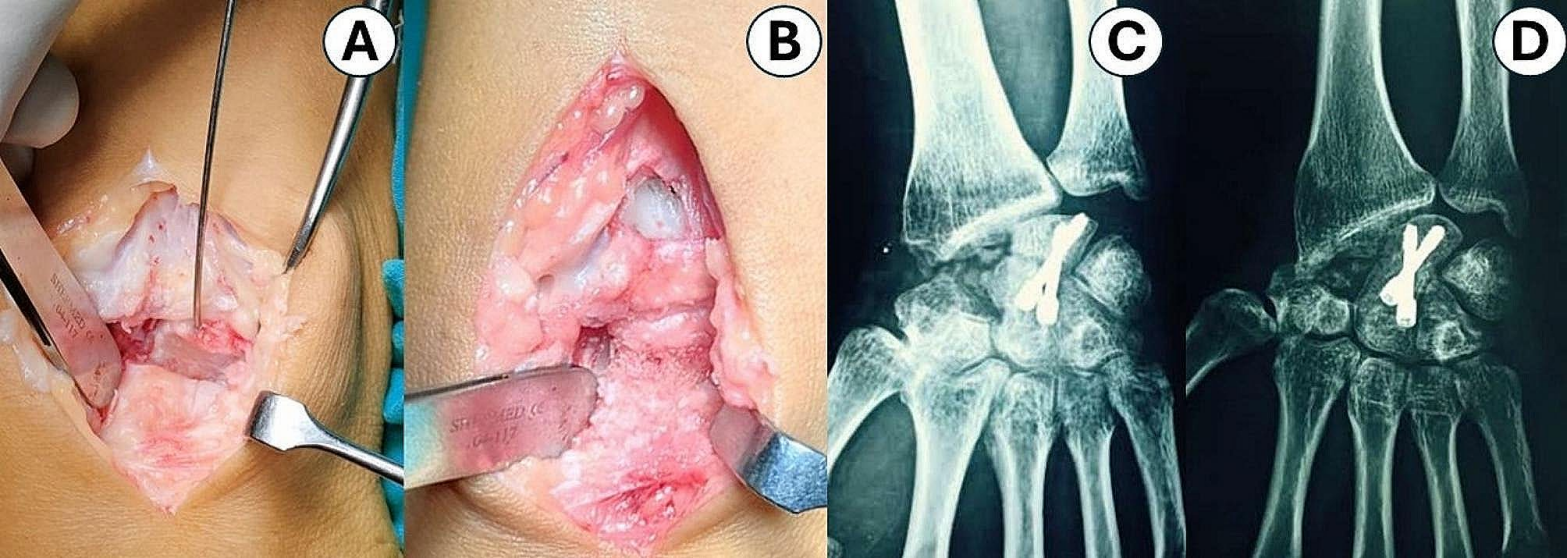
A: Intraoperative clinical photo demonstrating preparation of the LC articulation site, B: The LC site following fixation and bone grafting, C: Early postoperative wrist radiograph showing complete scaphoidectomy and LCF using two Herbert screws inserted in a retrograde manner; D: Wrist radiograph showing healing of the LCF site
